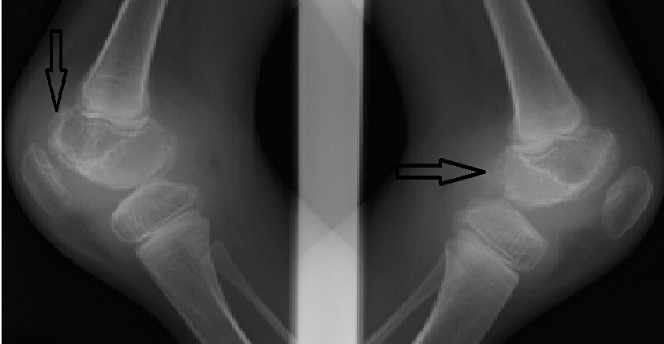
Enfant 15 ans, hémophile A sévère. Radiographie comparative en incidence de profil des genoux. Irrégularité des corticales osseuses condyliennes fémorales bilatérales (flèches). Noter l'hypertrophie épiphysaire et le gonflement des parties molles des deux genoux
